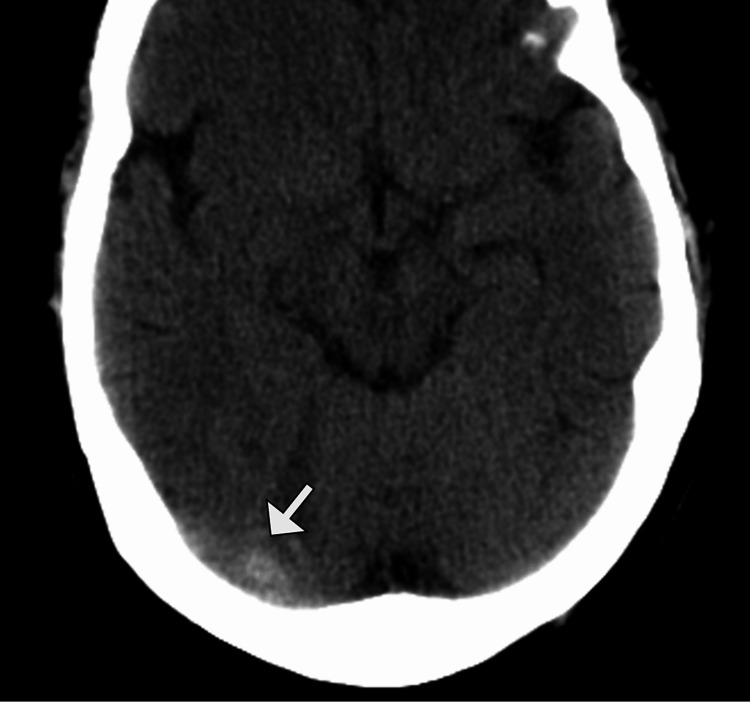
Noncontrast CT demonstrating right transverse sinus thrombosis Axial noncontrast computed tomography (CT) image of the brain shows a focal hyperdensity within the right transverse sinus (arrow), consistent with the dense sinus sign and suspicious for acute transverse sinus thrombosis.
